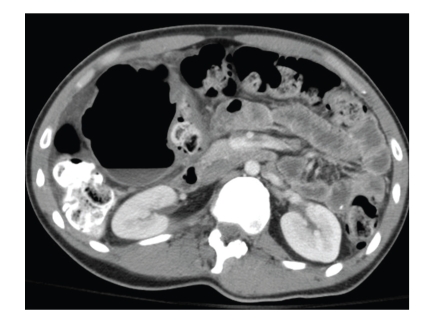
Computed tomography of the abdomen demonstrating the cyst containing an air-fluid level.
